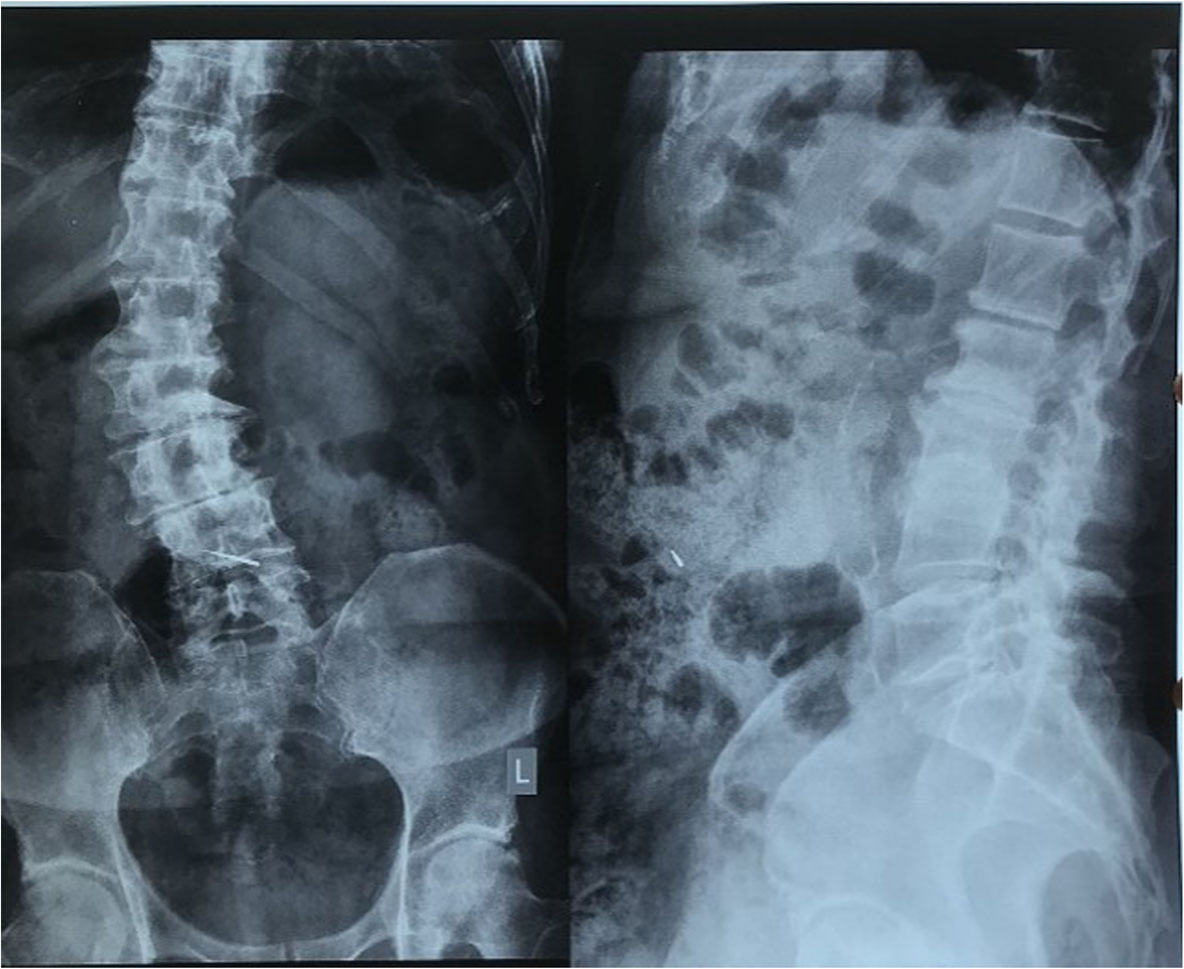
Abdominal X-ray image showing the presence of a linear pointed radiopaque foreign body in the anterior aspect of mid abdomen (L4 level)
